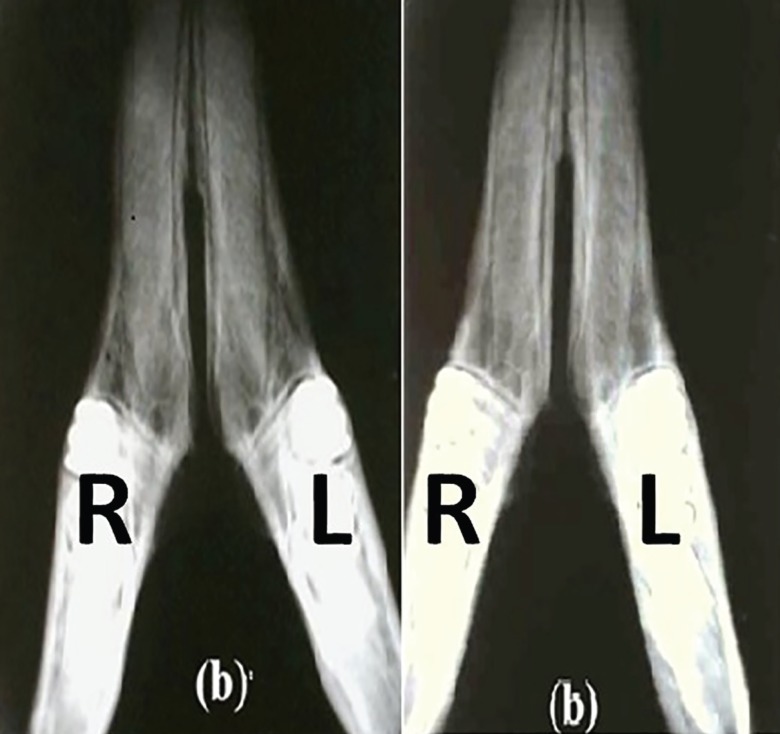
Radiographic observation on day of injury. a) Left R: the defect filled with fibrin glue; Left L: the control defect, b) Right R: the defect filled with fibrin glue; Right L: the defect filled with autologous bone graft after 4 weeks.
